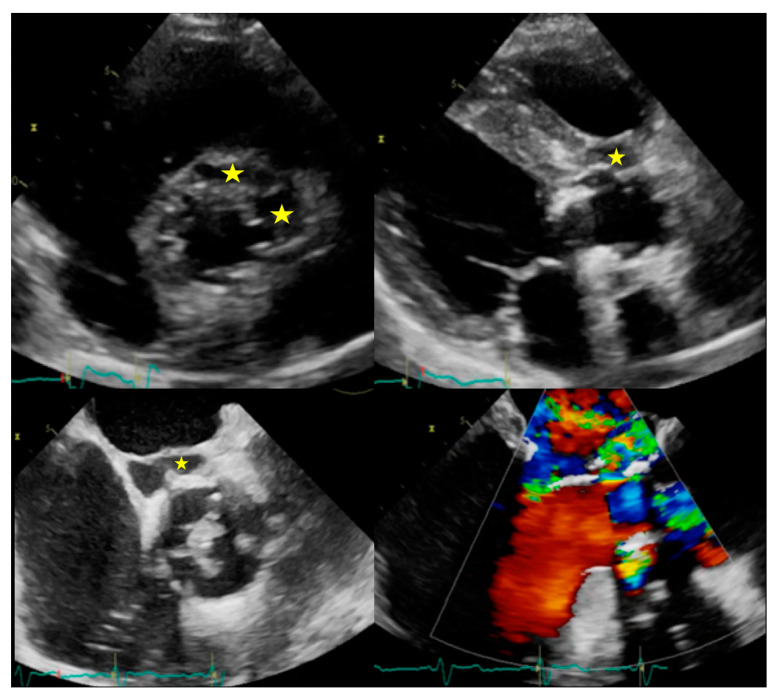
Complications of IE. Top: Example of peri-aortic abscess (stars) with large anechoic cavity surrounding the biological prosthesis (left: TTE with parasternal short axis view, right: TTE with parasternal long axis view). Bottom: Example of peri-aortic pseudoaneurysm (star) with large cavity communicating with cardiovascular lumen (left: TOE short axis view showing large vegetation on prosthesis cusps, right: TOE log axis view with color Doppler showing flow into the perivalvular cavity).
